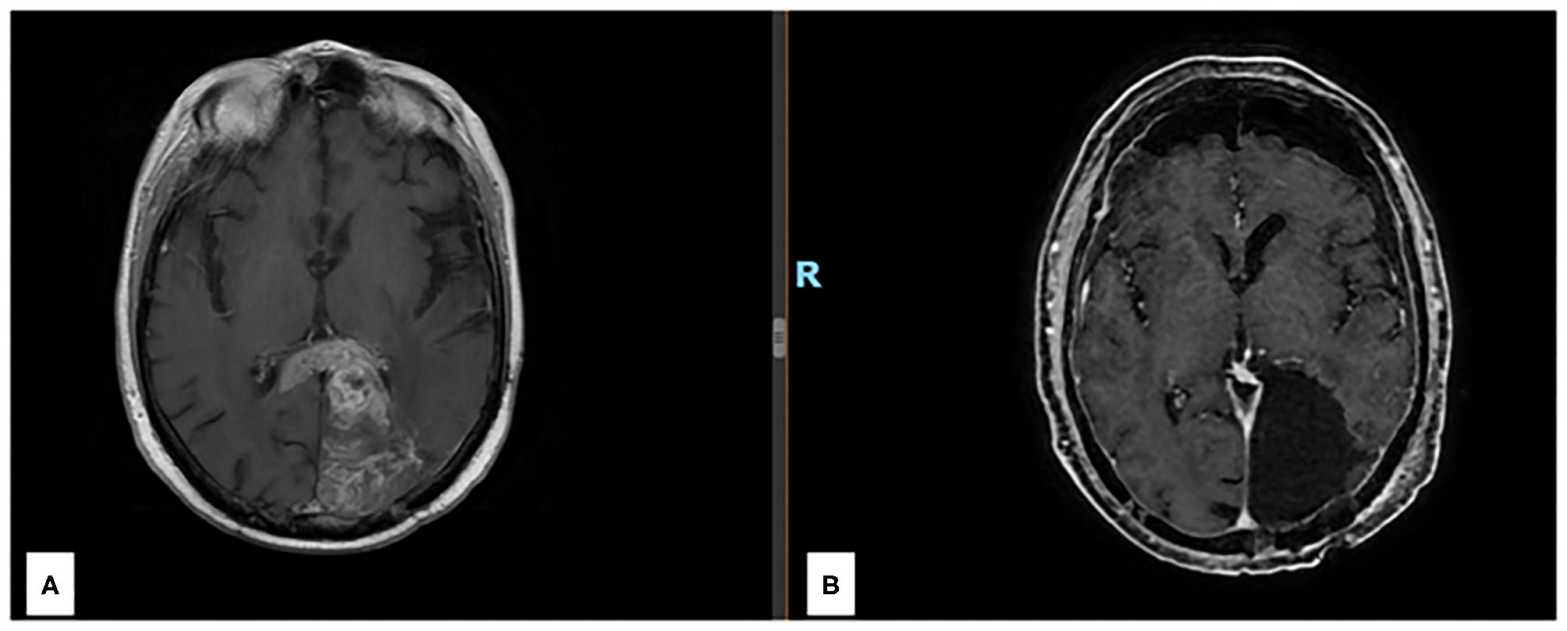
(A) Preoperative axial section of a T1 w MRI after gadolinium injection revealing a left parieto-occipital recurrent lesion with infiltration of the splenium of the corpus callosum. (B) Postoperative axial section of a T1 w MRI after gadolinium injection revealing the complete resection of the enhancing nodule.
